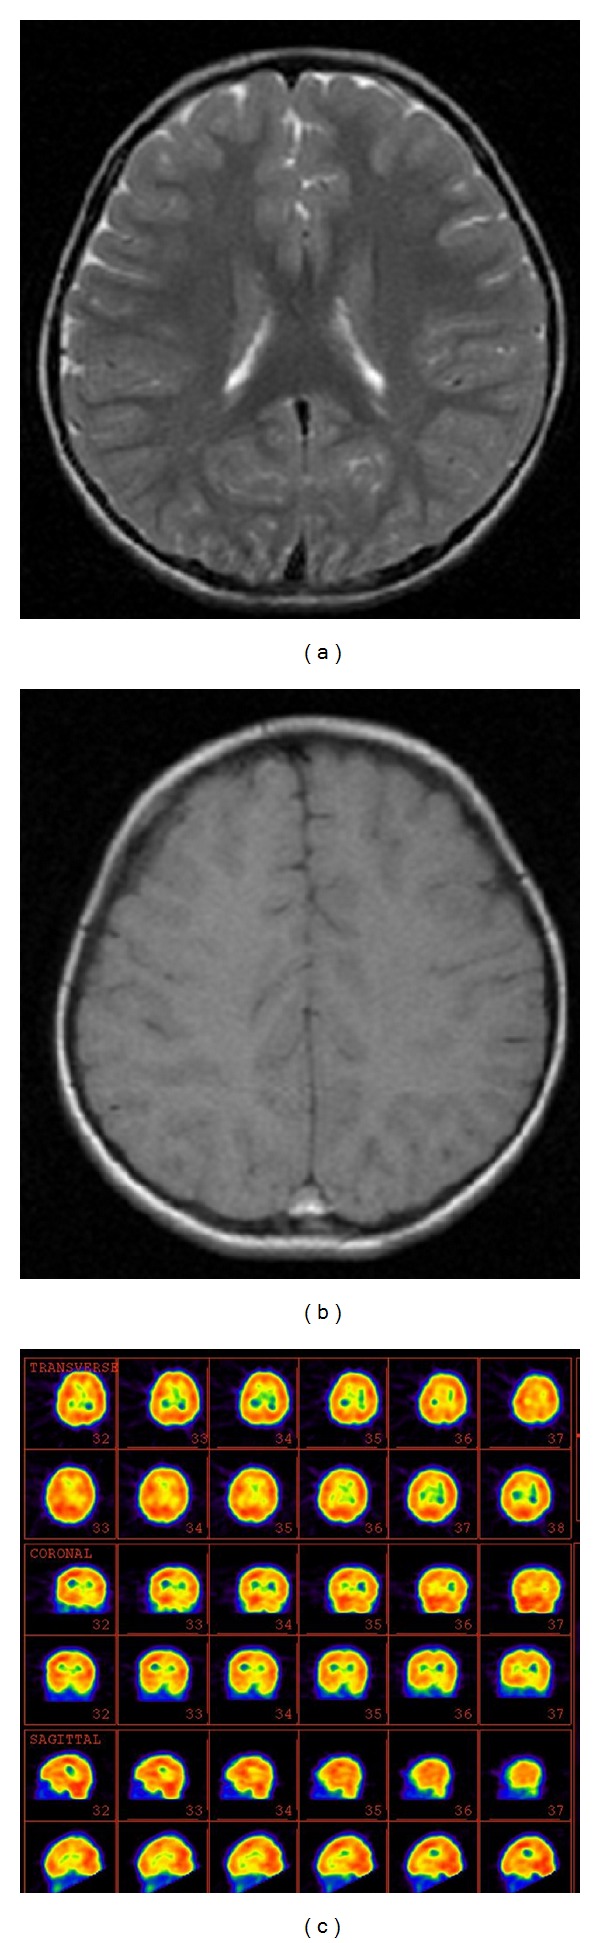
((a), (b)) The MRI images of a 6-year-old boy with Landau-Kleffner syndrome (LKS). (c) 99mTc-ECD brain perfusion SPECT image showed hypoperfusion in the left inferior frontal, left temporal, and also left thalamic regions. The upper rows indicate transverse 99mTc-ECD SPECT images. The middle rows indicate coronal 99mTc-ECD SPECT images. The lower rows indicate sagittal 99mTc-ECD SPECT images.
